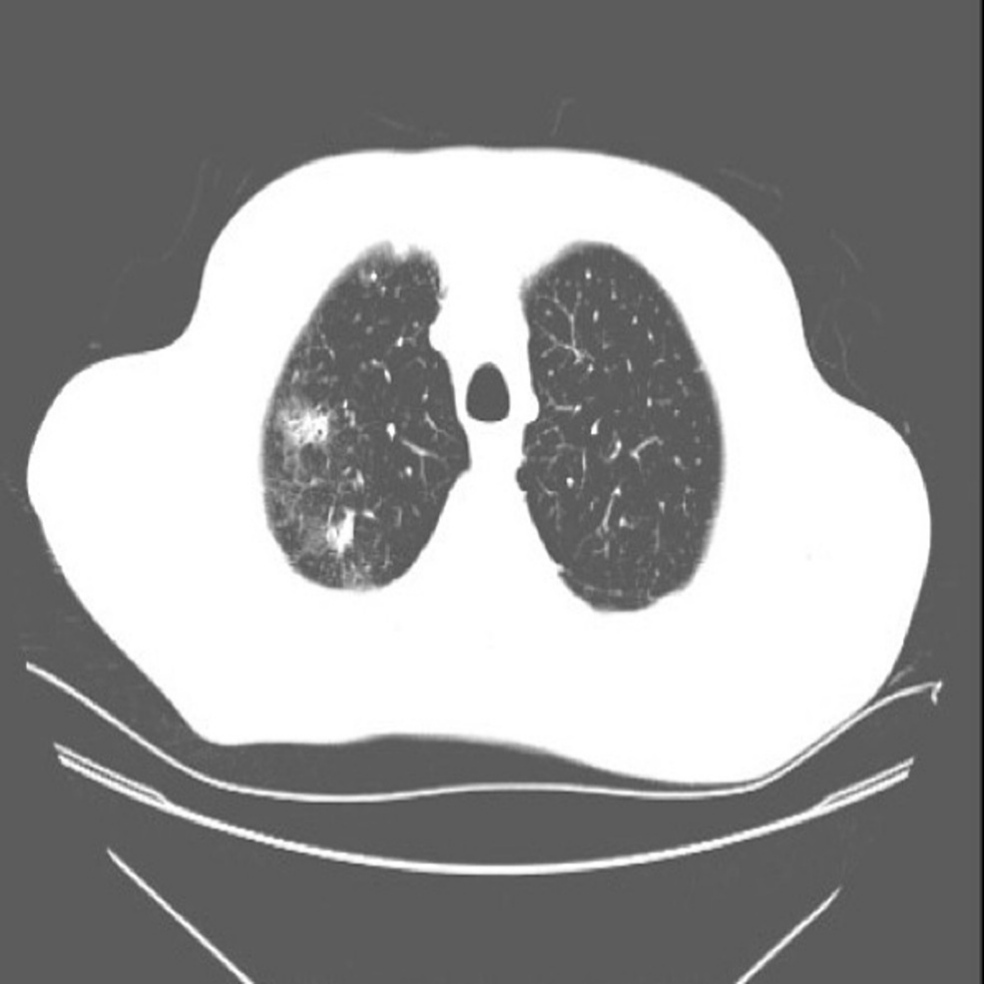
Reticulonodular infiltrates in the right upper lobe on CT
